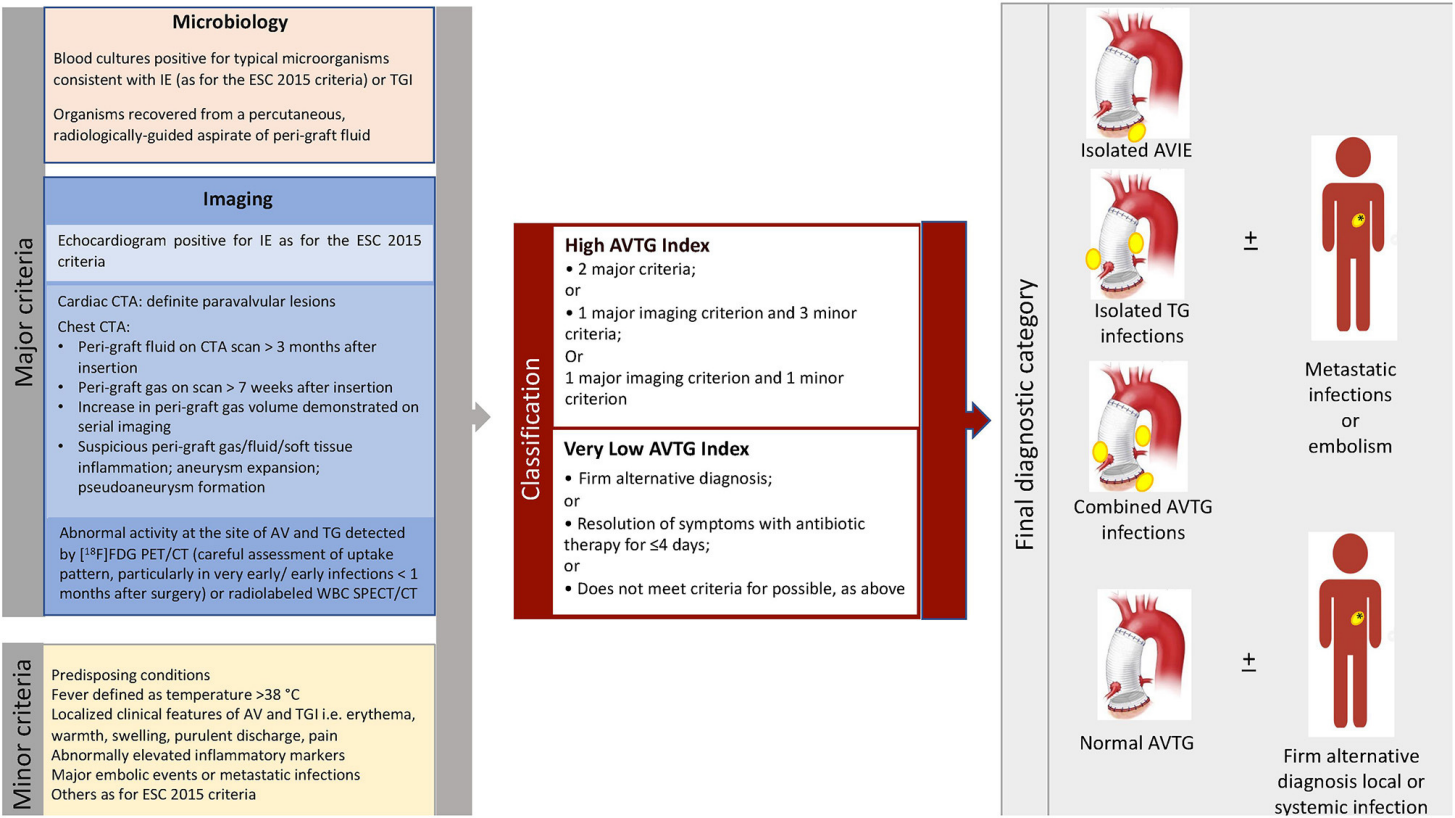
Summary of the major and minor criteria for the diagnosis of aortic valve and aortic tube graft infection proposed (aortic valve tube graft criteria, left panel), interpretation (middle panel), and final classification of patients based on their application (right panel). AVTG, aortic valve tube graft; CTA, CT angiography; [^18^F]FDG, 18-fluoride-fluorodeoxyglucose; IE, infective endocarditis; SPECT, single-photon emission CT; TGI, tube graft infection.
